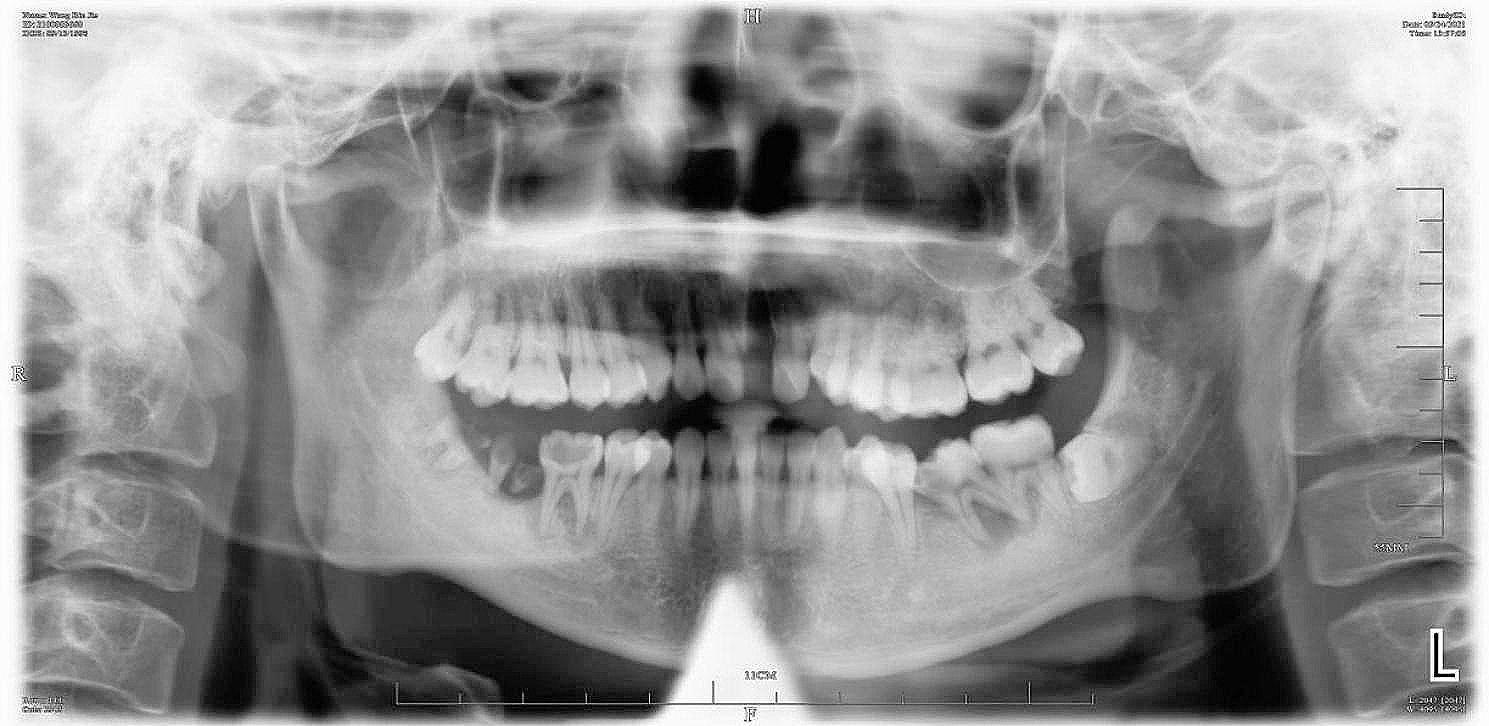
Panoramic radiographs on the first visit
